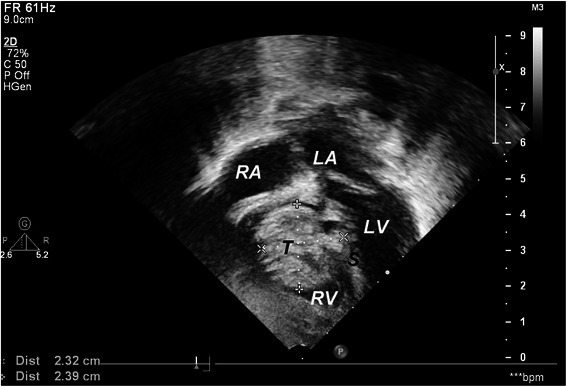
Two-Dimensional echocardiogram (transthoracic) showing well-defined tumour mass (T) attached to the interventricular septum (S) adjacent to the tricuspid valve on the right ventricle cavity. (LV-Left Ventricular Cavity, RA-Right Atrium, LA-Left Atrium, RV-Right Ventricular Cavity).
